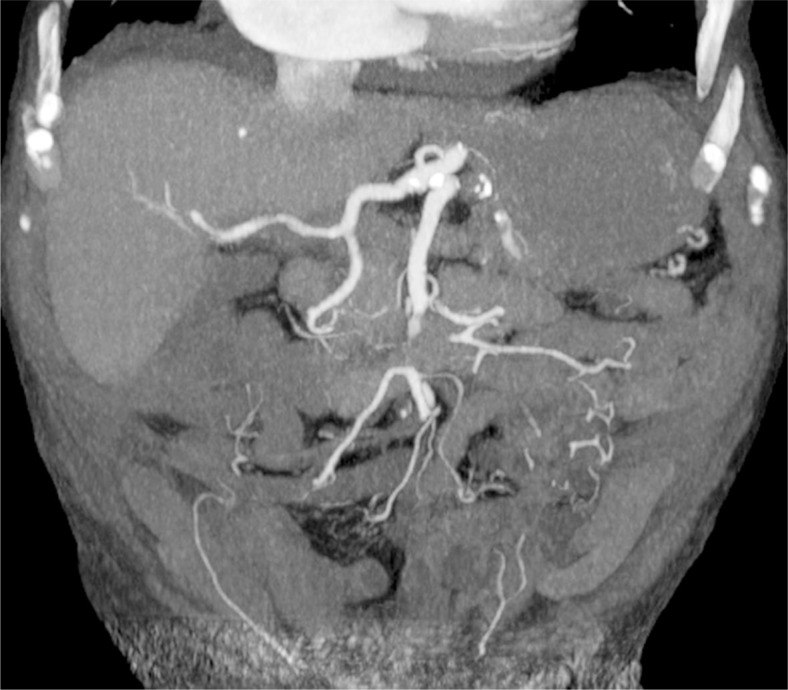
Coronal MIP reconstructions of CTA, revealing a segmental, occlusive acute embolism of the mid portion of SMA stem.
